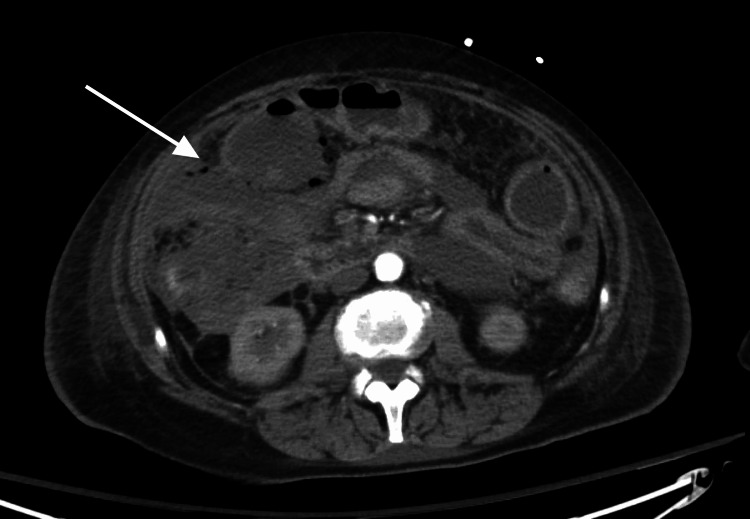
CT imaging demonstrated hydropneumoperitoneum, pneumatosis intestinalis, and bowel wall thickening, findings consistent with bowel ischemia and perforated viscus (arrow).
